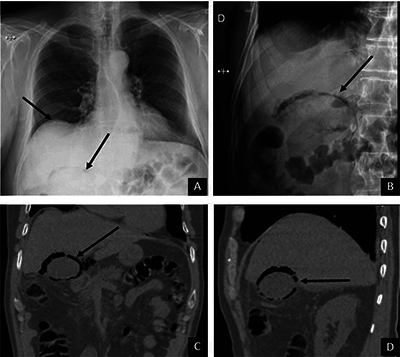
Chest radiography shows a pneumoperitoneum and an AC in the right hypochondrium (A). A supine abdominal X-ray confirmed the AC at the level of the gallbladder bed (B). Unenhanced abdominal CT scan shows air within or next to the gallbladder wall (C and D) related to emphysematous cholecystitis.
